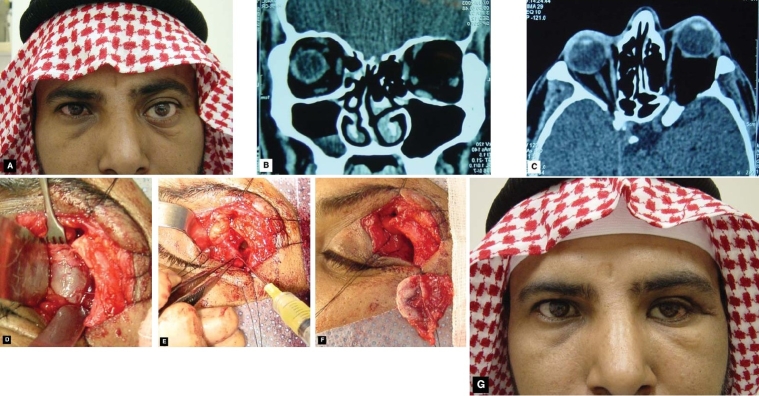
External photograph of a 34-year-old male who presented with a gradually progressive painless left proptosis.(A) Computed tomography scan revealed left eye proptosis and cystic lesion behind left eye globe pushing the optic nerve medially and expansion of left orbit and erosion of the roof, floor and lateral wall, (coronal B; axial C). Exposure of the deep dermoid cyst through lateral orbitotomy incision (D), controlled aspiration of the cystic fluid (E), and complete removal of the wall of the cyst from the apex of left orbit. (F) Two-week post-operative photograph of the same patient showing slight enophthalmos on the left side. (G)
